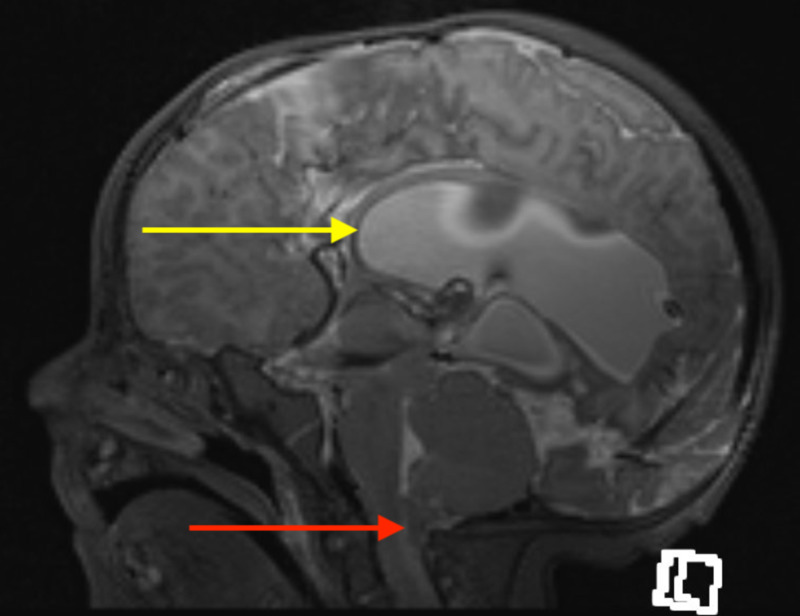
T2 sagittal MRI showing a Chiari II malformation (red arrow) and enlarged ventricles (yellow arrow) MRI: magnetic resonance imaging
